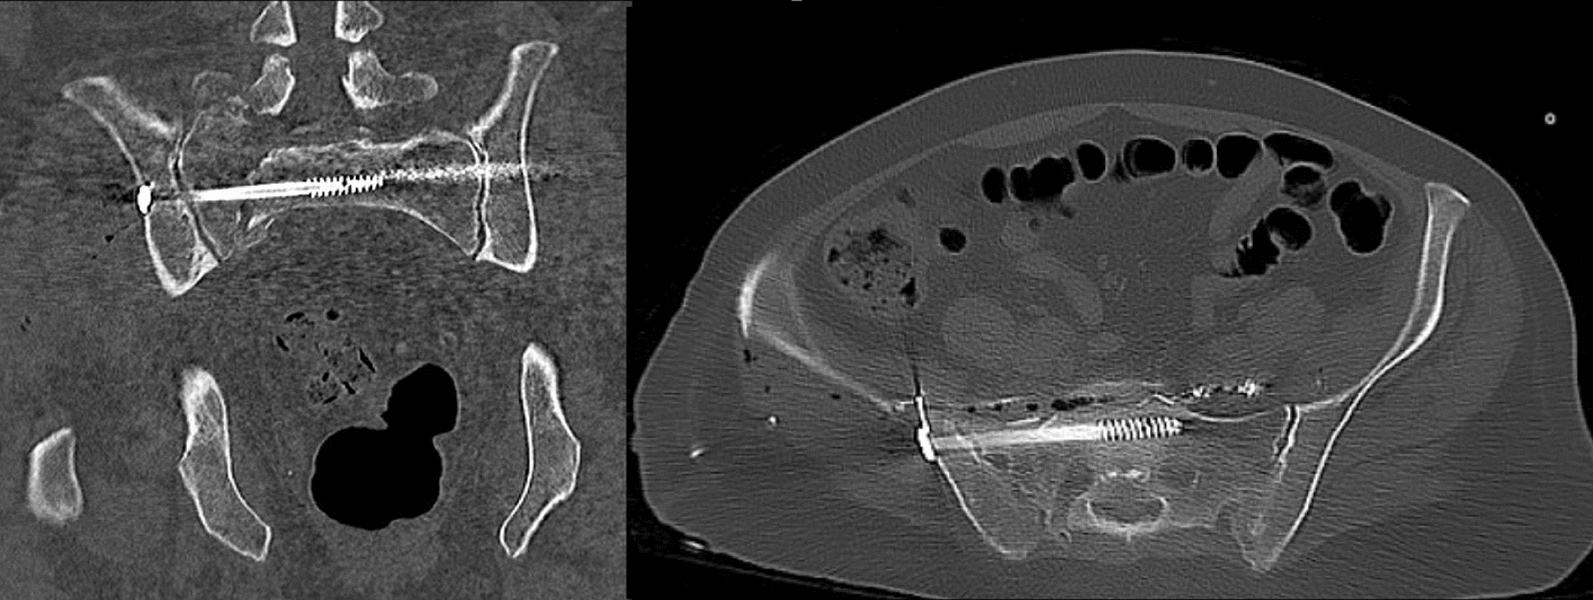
Postoperative CT scan confirming the correct position of the ISs
